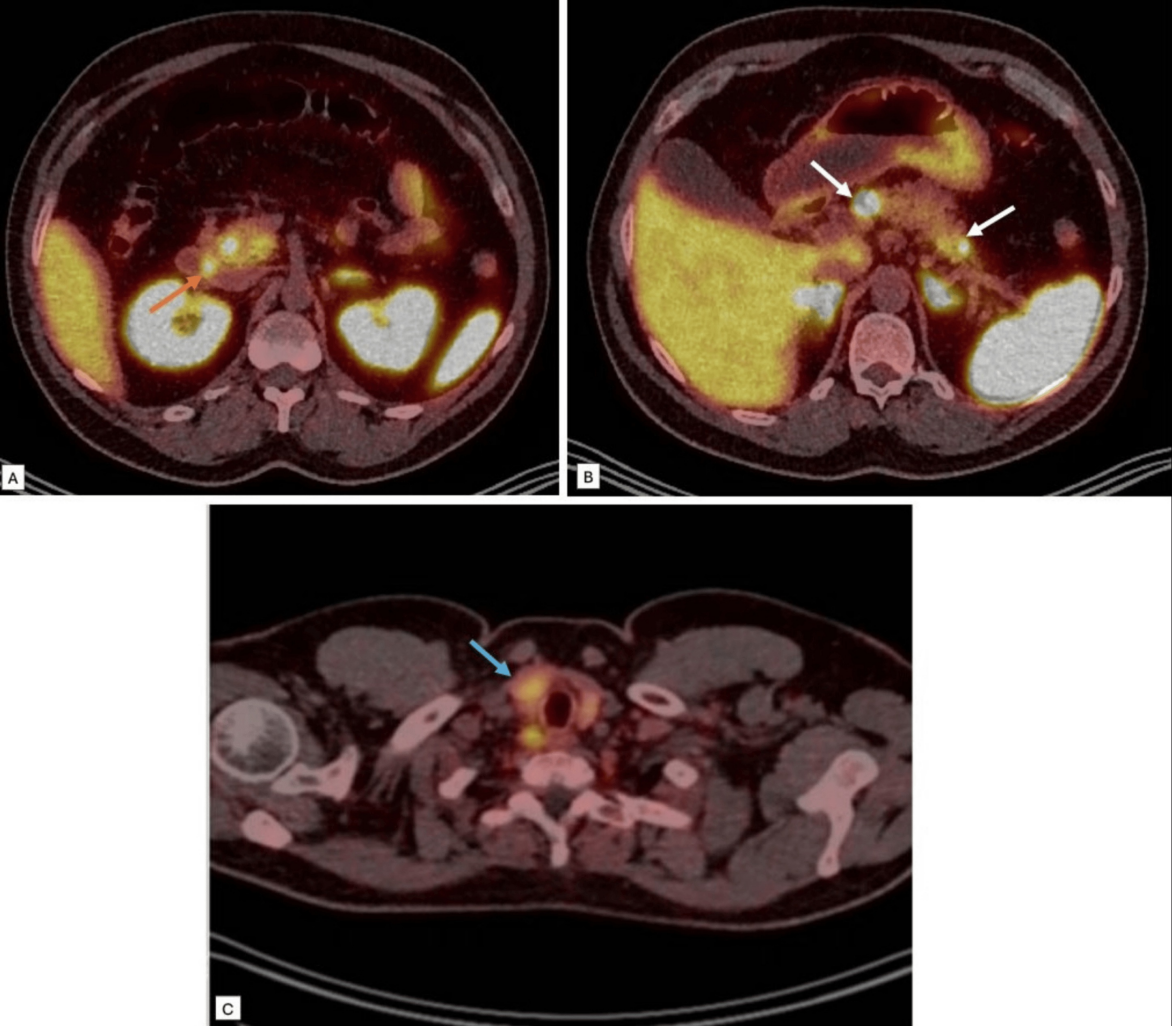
Ga68 DOTA-TATE whole body PET-CT. Multiple foci of intense tracer uptake in the pancreas (white arrows in image (B)) and a focus of uptake in the duodenum (orange arrow in image (A)), and the right inferior parathyroid adenoma (blue arrow in image (C)). PET: positron emission tomography.
